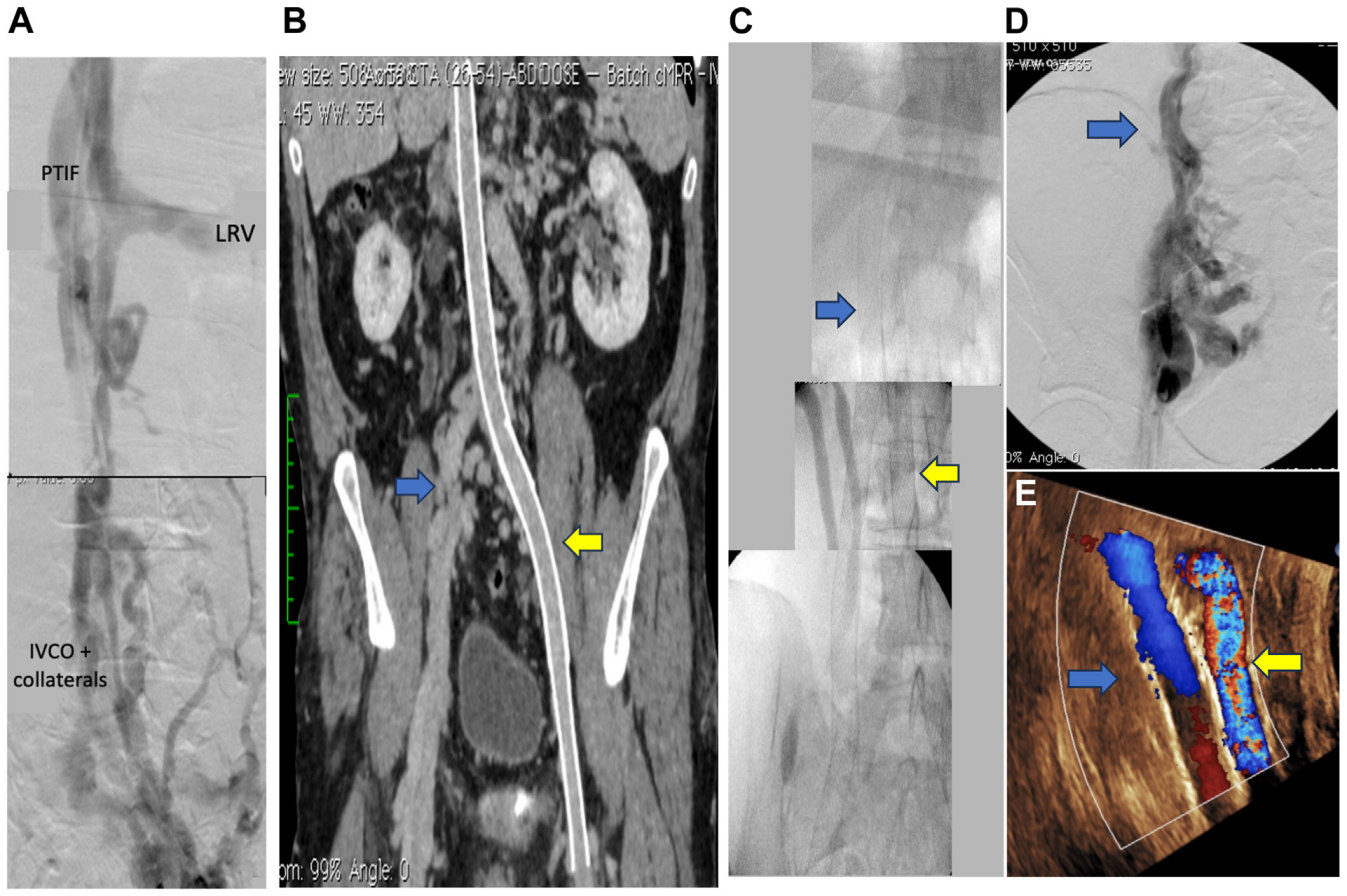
A, Angiogram showing post-thrombotic left lower extremity with iliac vein occlusion and caval outflow. B, Computed tomography scan of left inferior vena cava occlusion (IVCO) stenting. The *blue arrow* indicates right common iliac vein occlusion with pelvic collateral veins; and the *yellow arrow* shows left iliocaval stent placement. C, The *blue arrow* shows the stented right common femoral vein and azygos vein, with drainage into the superior vena cava (SVC); the *yellow arrow* depicts the stented left common femoral vein and iliac vein, with drainage into the suprahepatic inferior vena cava. D, Venogram showing right-sided pelvic collateral vessels draining into the azygous vein before azygos vein stenting (AVS). E, Duplex ultrasound (image rotated) at 12 years showing patent right azygos (*blue arrow*) and left caval (*yellow arrow*) stents. *LRV,* Left renal vein; *PTIF,* post-thrombotic intraluminal fibrosis.
